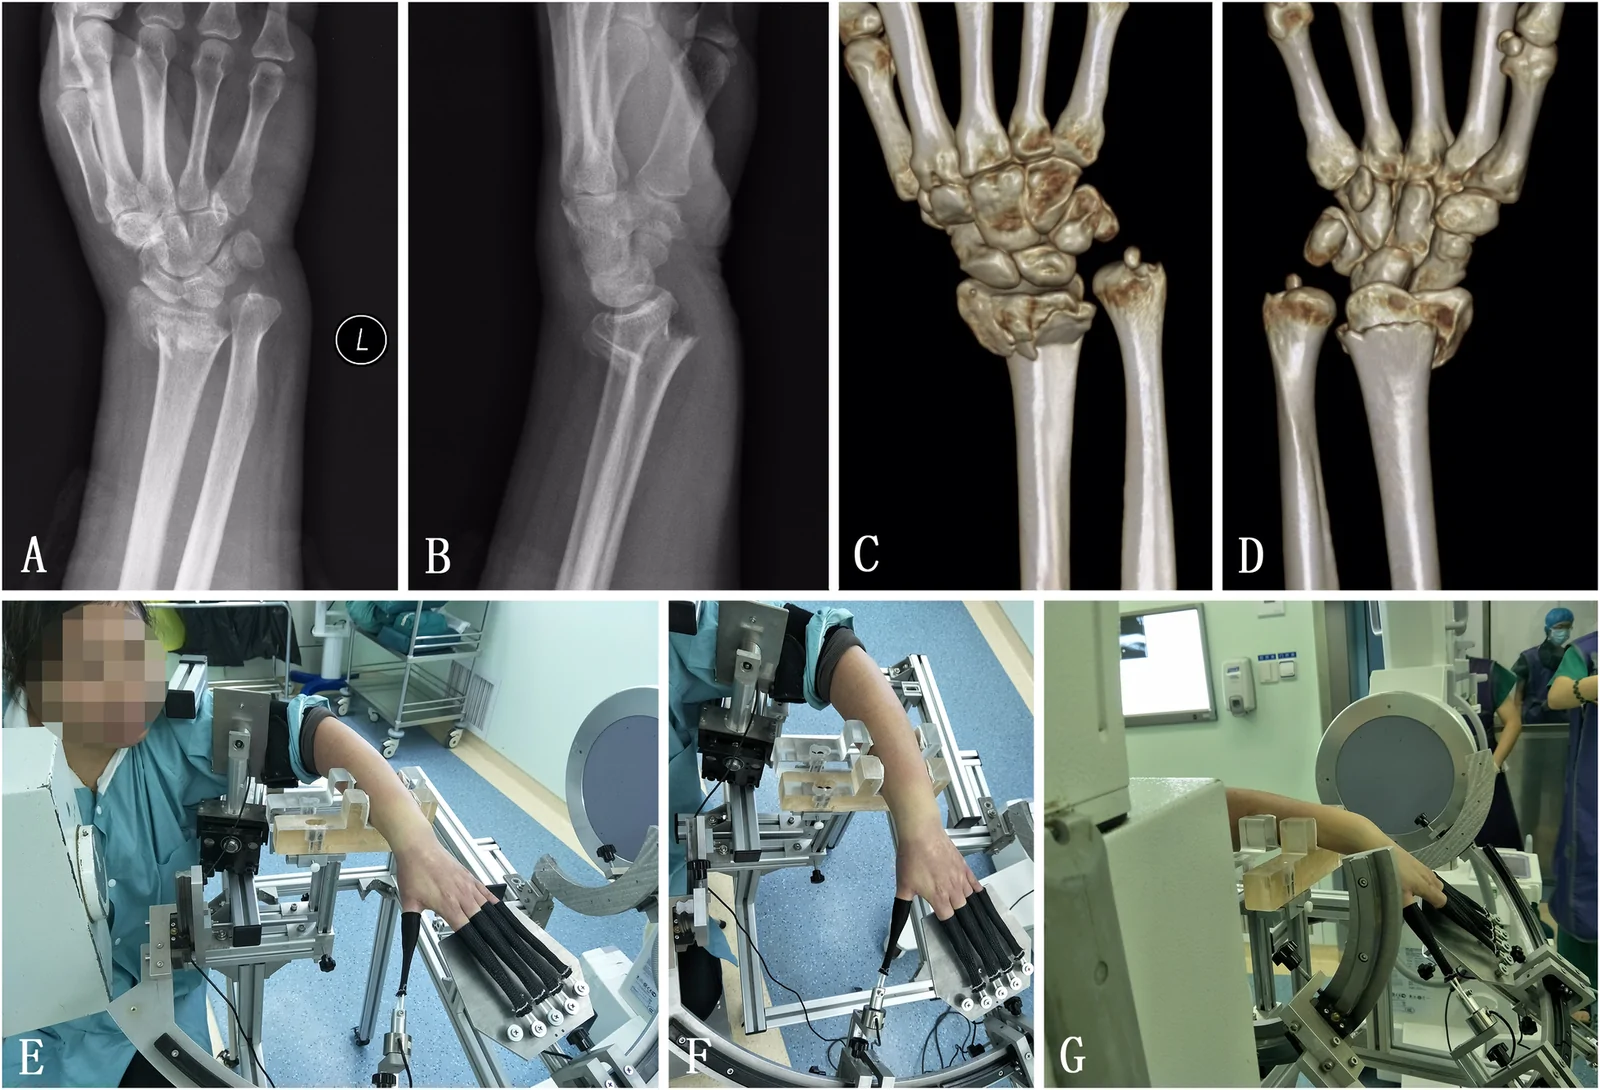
A 66-year old female fell down from a standing height and suffered from fractures of left distal radius and ulnar styloid fractures. (A–D) The x-ray and CT views of the fractures. (E–G) The position of patient and the injured upper limb.
